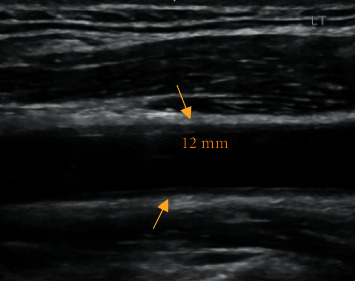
Longitudinal ultrasound view showing a noticeable improvement in LCC wall thickening (arrows) after corticosteroid therapy, as compared to Figure 1(a). 0.12 cm relates to vessel wall thickness. ^∗^Dimensions not to scale. LCC : left common carotid.
